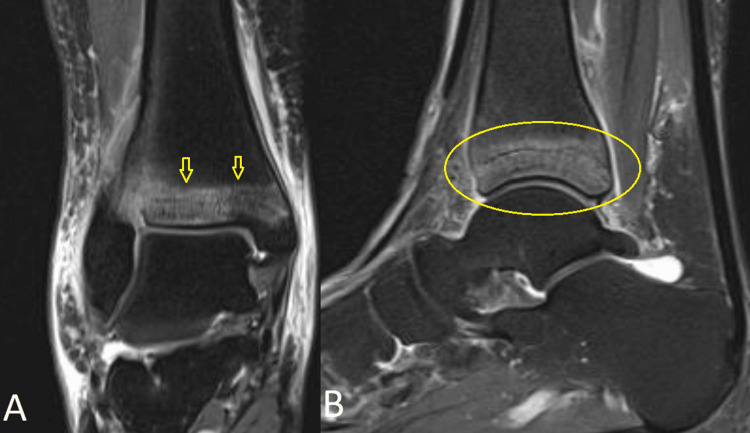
MRI of the right ankle in the (A) coronal and (B) sagittal fat saturated views showing an acute oblique non-displaced fracture of the distal tibial metaphysis with surrounding mild effusion of the subtalar and tibiotalar joints.
